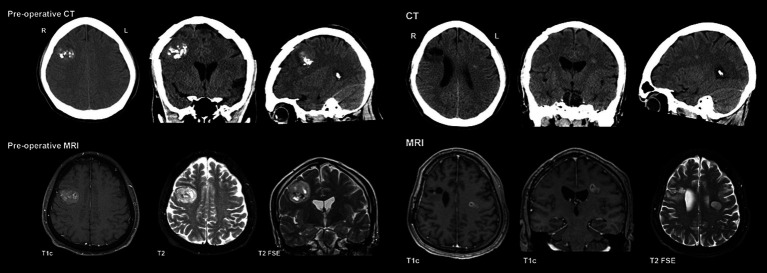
Preoperative CT/MRI for PLNTY & Preoperative CT/MRI for ependymoma.
